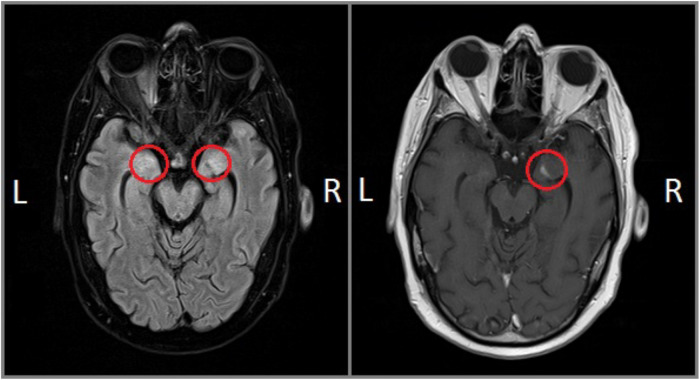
FF’s MRI scans: FLAIR (left) and T1-weighted gadolinium-enhanced (right) sequences.
